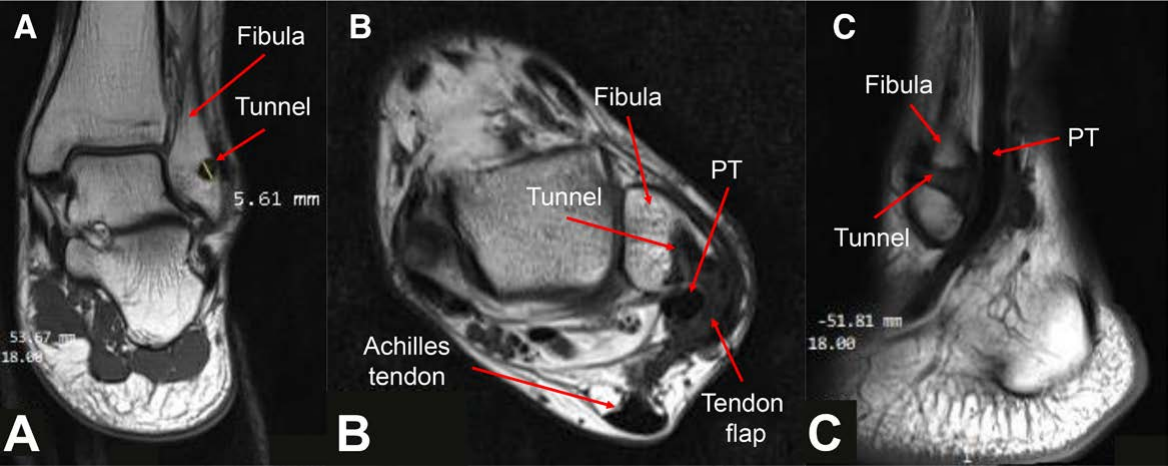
Postoperative MRI of the left ankle at 3-yr follow-up. (A) Coronal T1-weighted image demonstrating that the tendon flap has incorporated into the fibular tunnel; the tunnel diameter measures 5.61 mm. (B) Axial T1-weighted image showing the tendon flap incorporated into the fibular tunnel and the peroneal tendons in the correct anatomical position, without dislocation. (C) Sagittal T1-weighted image confirming the tendon flap incorporated into the fibular tunnel. MRI = magnetic resonance imaging, PT = peroneal tendon.
